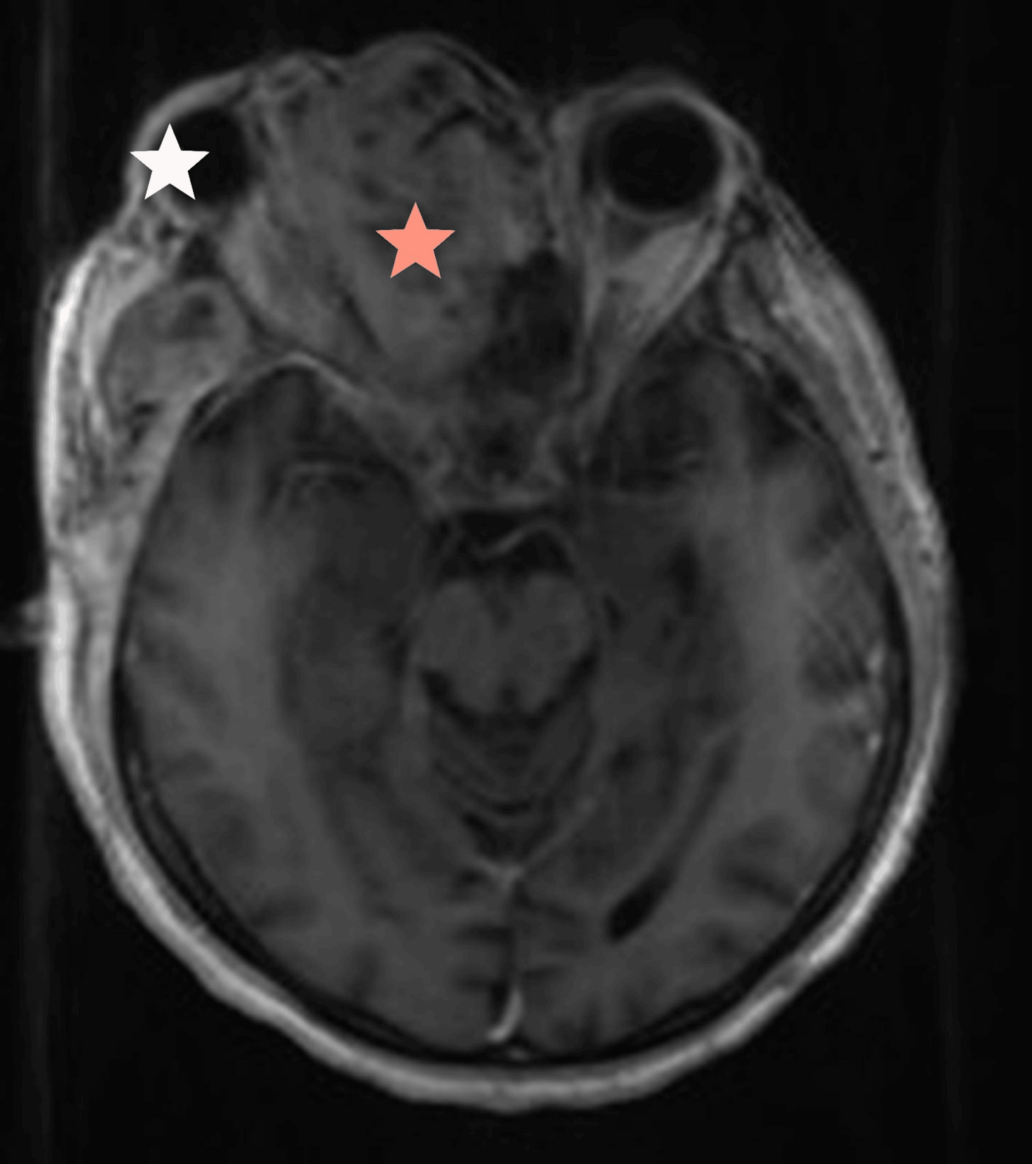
Axial T1-weighted MRI post contrast shows enhancement of the lesion (red star) with clear mass effect on the right orbital structures and proptosis (white star).
